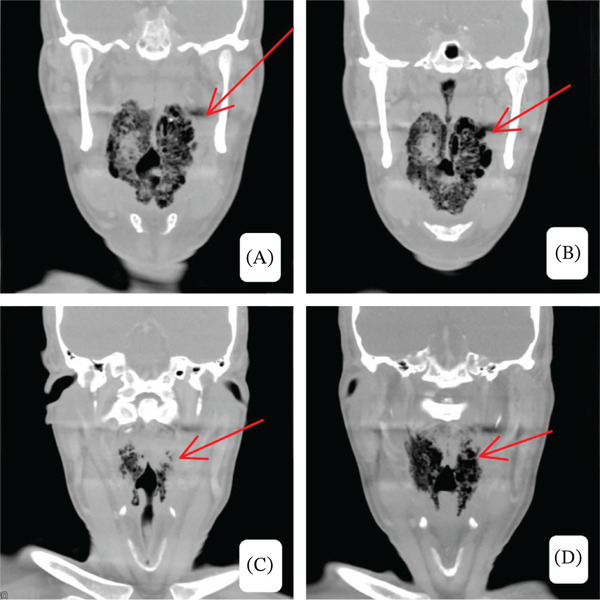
(A–D) Coronal contrast‐enhanced CT images at multiple levels demonstrate central and submucosal gas locules within the palatine tonsillar parenchyma, diffuse tonsillar enlargement, and surrounding inflammatory changes, consistent with emphysematous tonsillar infection. There is severe airway narrowing reflecting the critical compromise of the upper airway.
